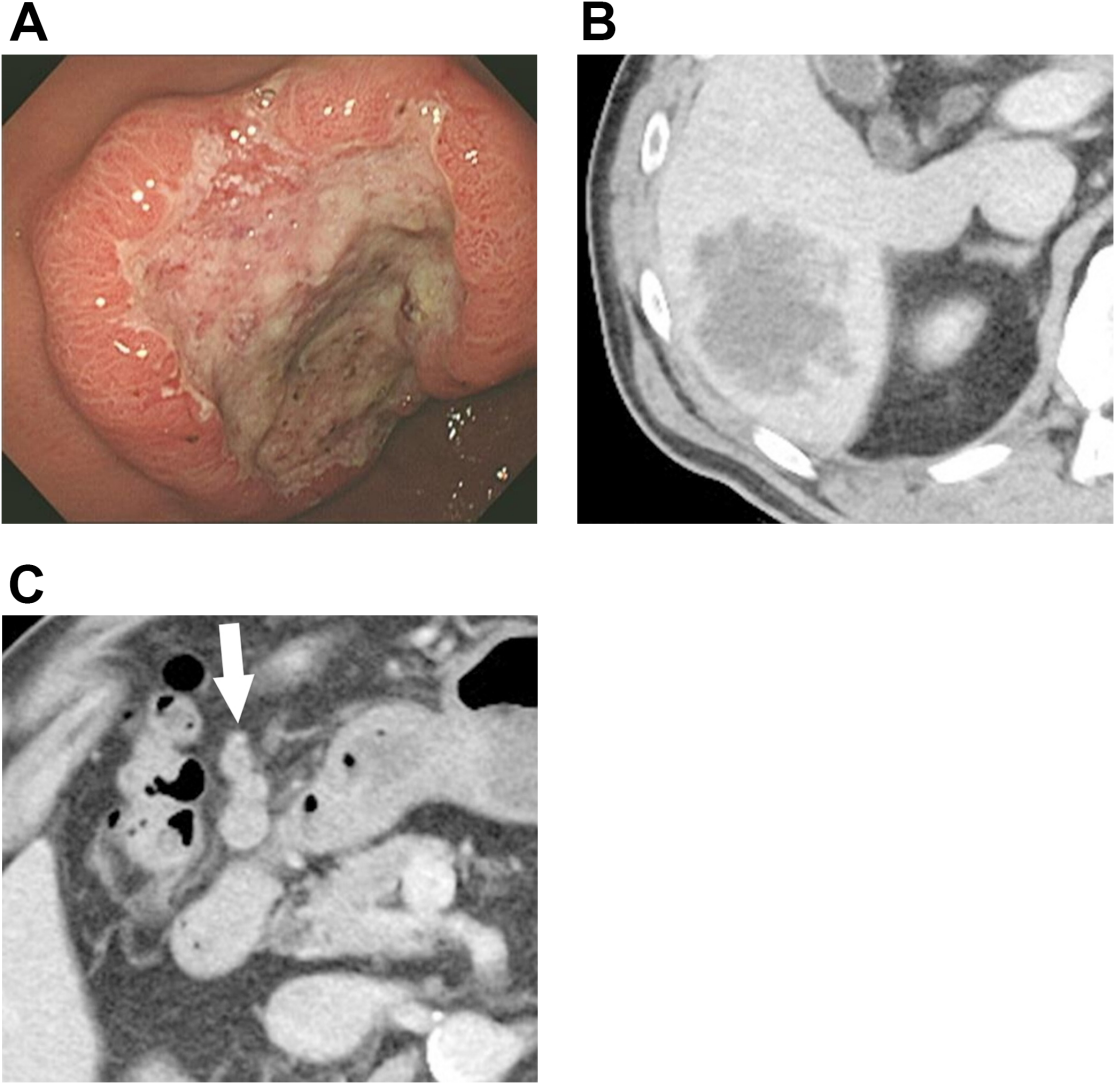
Findings from pre-chemotherapy examinations. (A) Endoscopy reveals a type 2 lesion, approximately 5 cm in diameter, located on the lesser curvature of the antrum. (B) Initial CT reveals an approximately 6-cm metastatic lesion in liver segment six with irregular margins and heterogeneous internal density. (C) At the initial presentation, multiple enlarged perigastric lymph nodes are observed, suggestive of lymph node metastasis (white arrow). CT, computed tomography.
